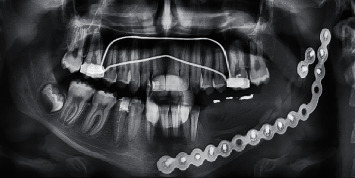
A radiographic image after denture delivery.
